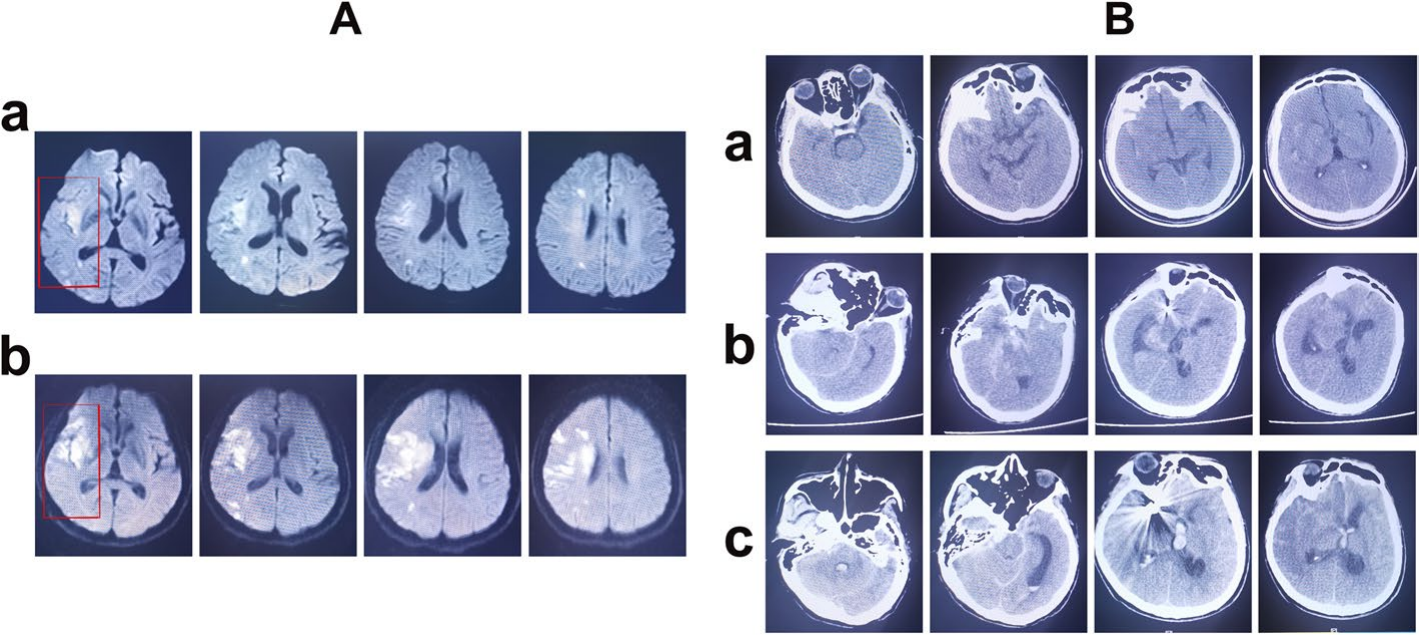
Magnetic resonance and computed tomography images. A Panel a: Diffuse-weighted magnetic resonance imaging sequence acquired on the day of admission. Panel b: Diffuse-weighted magnetic resonance imaging sequence acquired on the day after admission. The rectangular area displays a cerebral infarction lesion located in the left watershed region. B Panel a: Computed tomography image taken on the day of admission. Panel b: Computed tomography image taken on the day the patient suffered a new acute subarachnoid hemorrhage. Panel c: Computed tomography image taken during the final digital subtraction angiography
